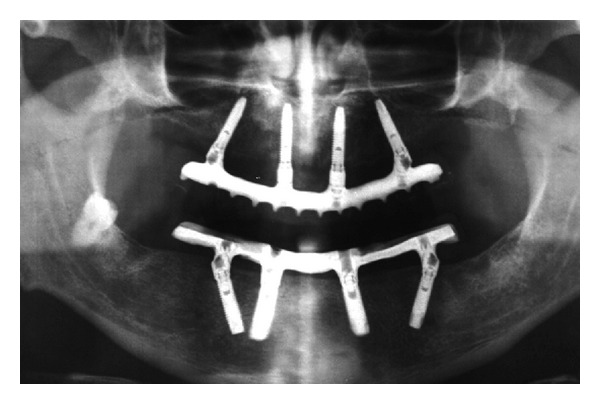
One year posttreatment orthopantomography.
